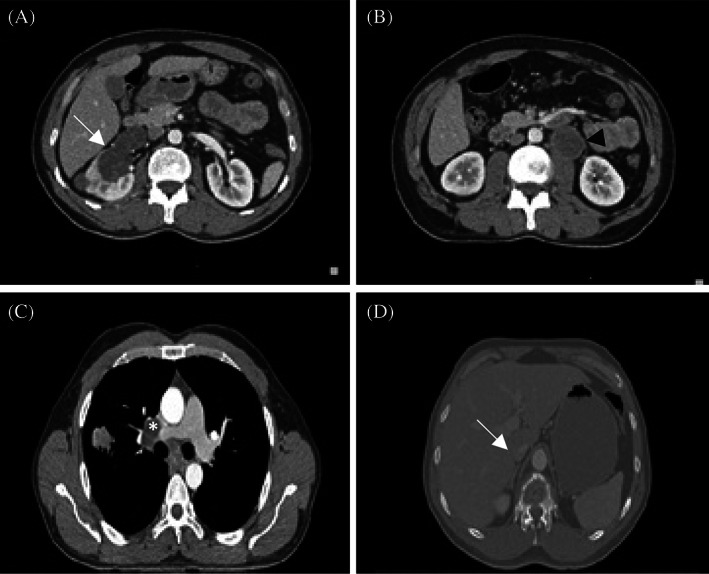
CT scan in December 2020 shows progression of all metastatic sites. (A) A 37 × 27 mm solid mass in medulla of the right kidney middle pole (arrow). (B) A 38 × 35 mm mass at the left paraaortic region (axial plane at the level of L2–L3) (arrow head). (C) Right lung mass, hilar, and mediastinal lymphadenopathies (axial plane at the level of T6). (D) Erosive lesion in T12 suggesting bone metastasis.
